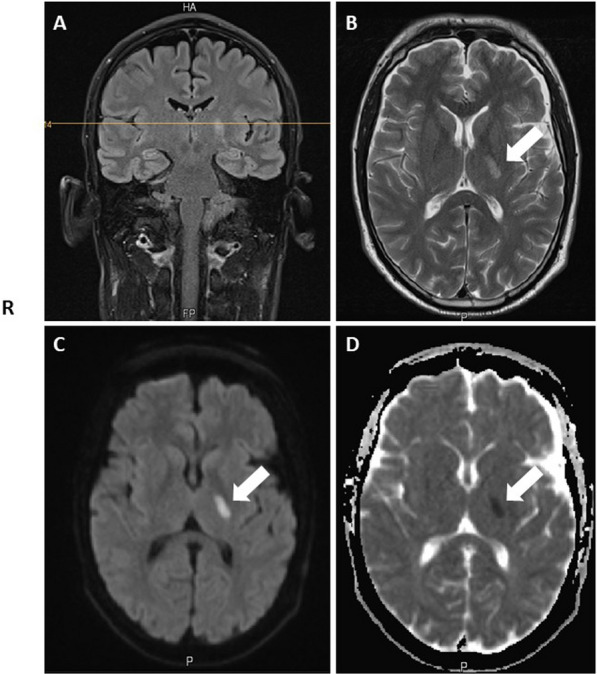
Cerebral MRT of reported patient. Coronar FLAIR (A), transversal T2 (B), diffusion weighted (C), and ADC map (D) imaging reveal a lacunar infarction in the left internal capsule (arrow). The horizontal line in (A) denotes the slice position of (B-D)
